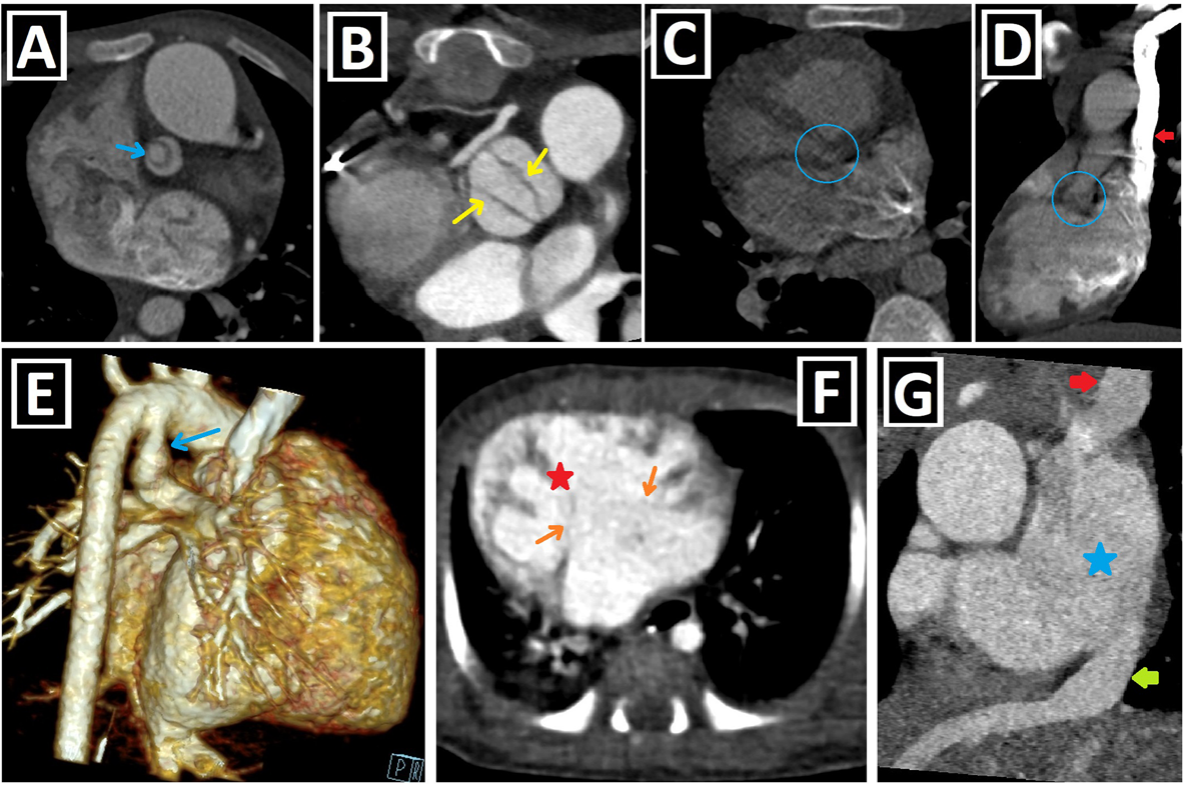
Right-sided lesions and single ventricular anomalies accompanying PLSVC. a, b Axial-oblique CT images indicate unicuspid (blue arrow, a) and bicuspid (yellow arrows, b) pulmonary valve in different patients with PLSVC. c, d Axial (c) and coronal-oblique reformatted (d) CT images depict severe pulmonary stenosis (blue circles) in a patient with PLSVC (red arrow). e–g 3D VRT (e), axial (f), and sagittal-oblique reformatted (g) CT images depict complex cardiac anomaly in a patient with PLSVC. This patient has pulmonary atresia with confluent right and left pulmonary arteries connected to the aorta with a large caliber PDA (blue arrow) (e). There is a single ventricle (red star) and a single atrioventricular valve (orange arrows) (f). PLSVC (red arrow) and IVC (green arrow) are draining into the common atrium (blue star) (g)
